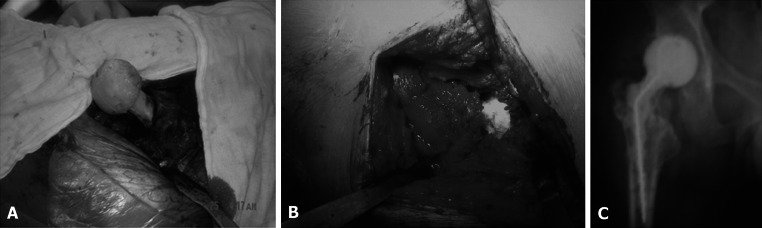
a Insertion prosthesis into femoral medullary canal. b Restoration of Antibiotic bone cement temporary functional prosthesis. c X-ray after implantation
